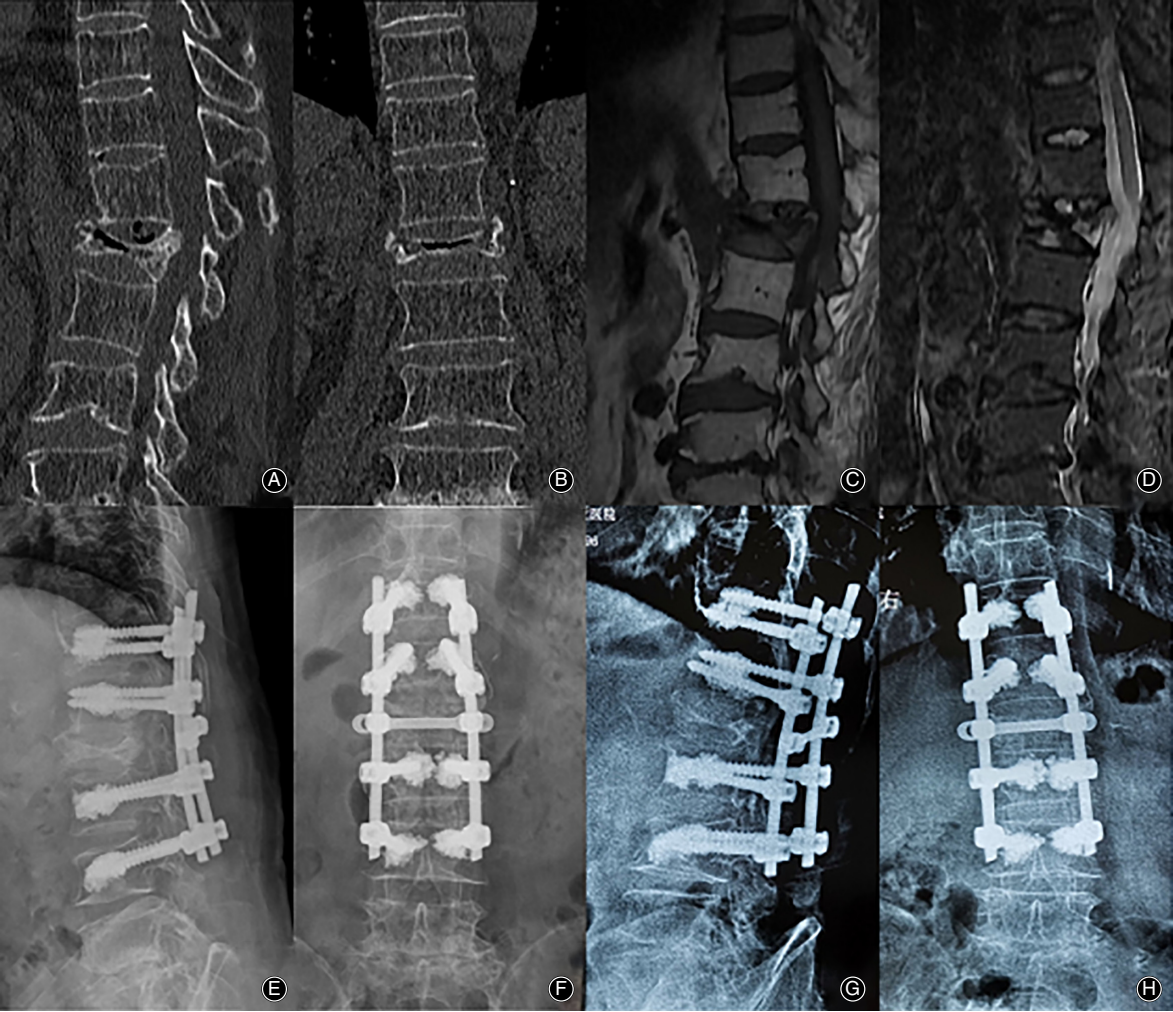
A 67‐year‐old‐female patient who underwent LSF for L_2_ Kummell disease. (A‐B) Preoperative CT scan showing a L_2_ burst fracture with IVC, and MRI showing low signal of the necrotic area and spinal cord compression on the T1(C) and T2‐weighted (D) images. Postoperative (E and F) and final follow‐up (G and H) X‐rays revealing good internal fixation position and satisfactory radiographic results.
